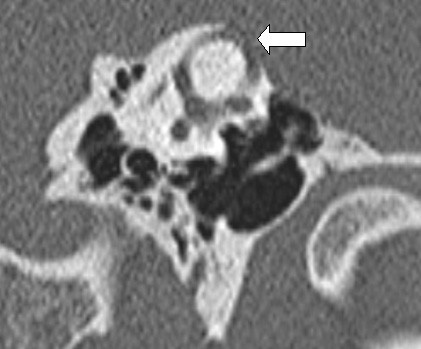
High-resolution CT scan showing a left superior semicircular canal dehiscence (arrow).
